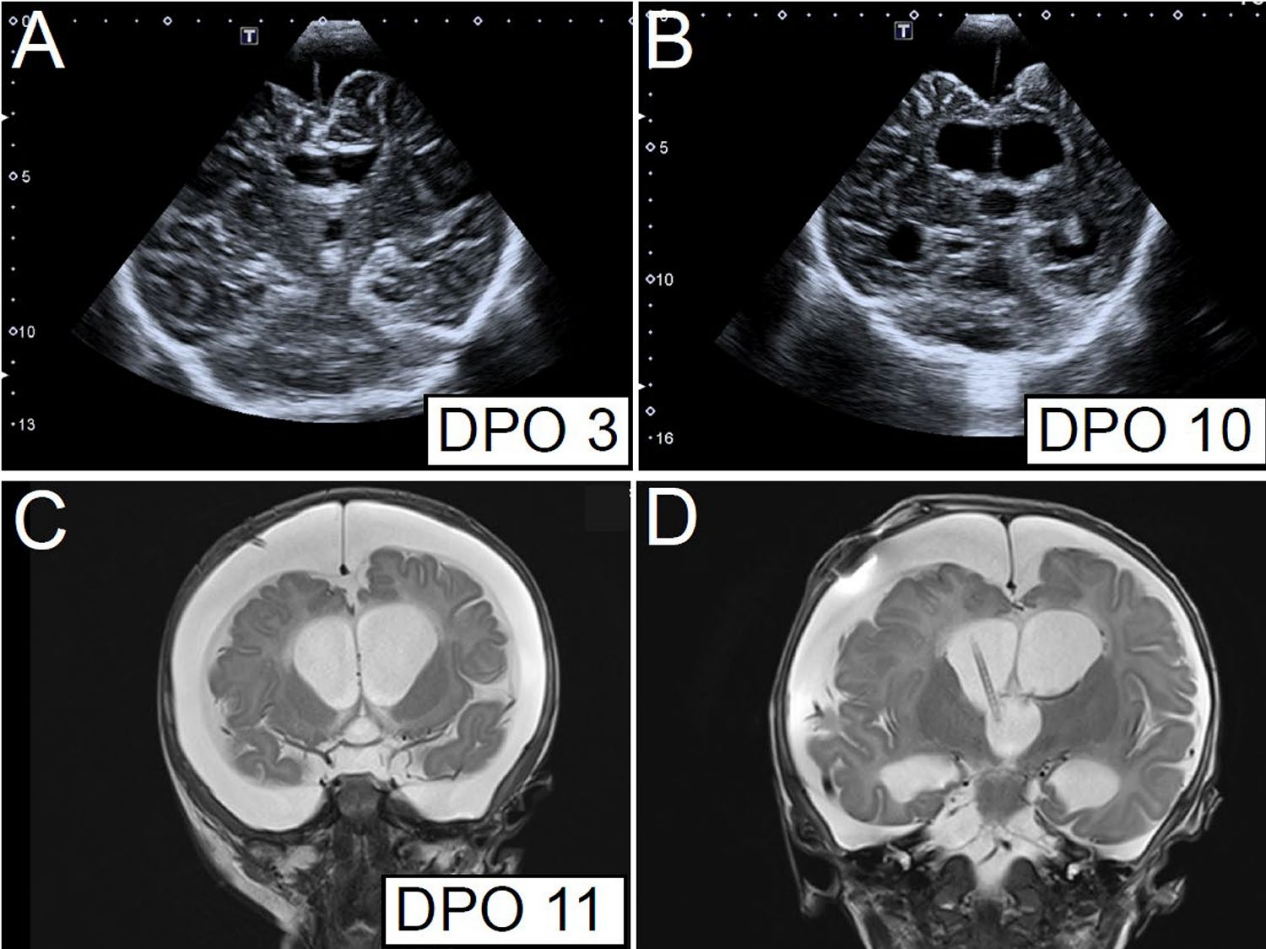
Follow-up imaging indicating dynamic development of hydrocephalus. A shows a follow-up coronal ultrasound scan after placement of a subduroperitoneal shunt with smaller but residual subdural hygroma and small ventricles. B demonstrates the enlargement of the supratentorial ventricles at the 10th postoperative day; C T2-weighted coronal MRI verifies these results one day later. As a consequence, a ventricular catheter was additionally placed and connected to the existing subduroperitoneal shunt system leading to decrease in CSF spaces, respectively, as shown in the coronal plane of the T2-weighted MRI (D)
